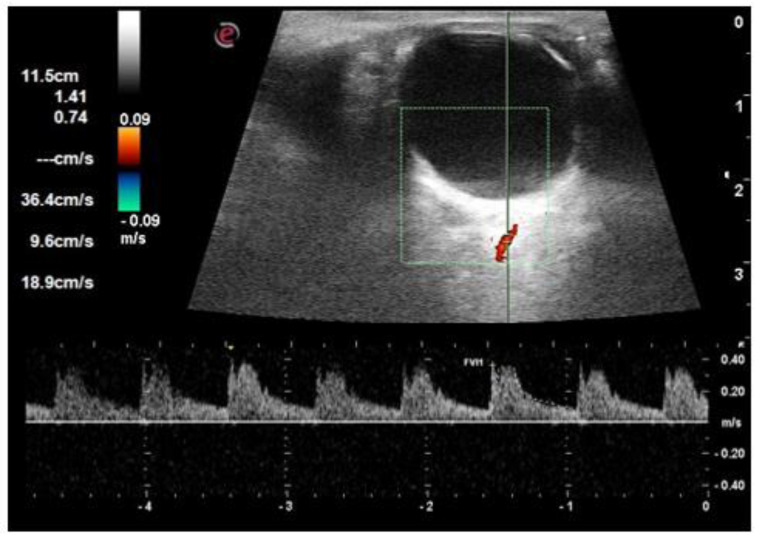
An orbital Doppler image of ophthalmic artery in a control subject shows a resistive index of 0.74.
